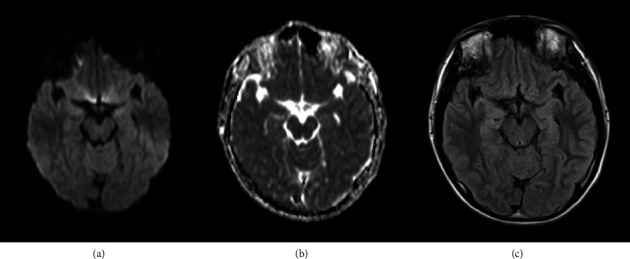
Normal axial magnetic resonance imaging (MRI) findings in the mesial temporal lobe on day 5 after symptom onset. (a) Axial diffusion-weighted imaging (DWI). (b) Axial apparent diffusion coefficient imaging. (c) Axial fluid-attenuated inversion recovery (FLAIR) imaging.
